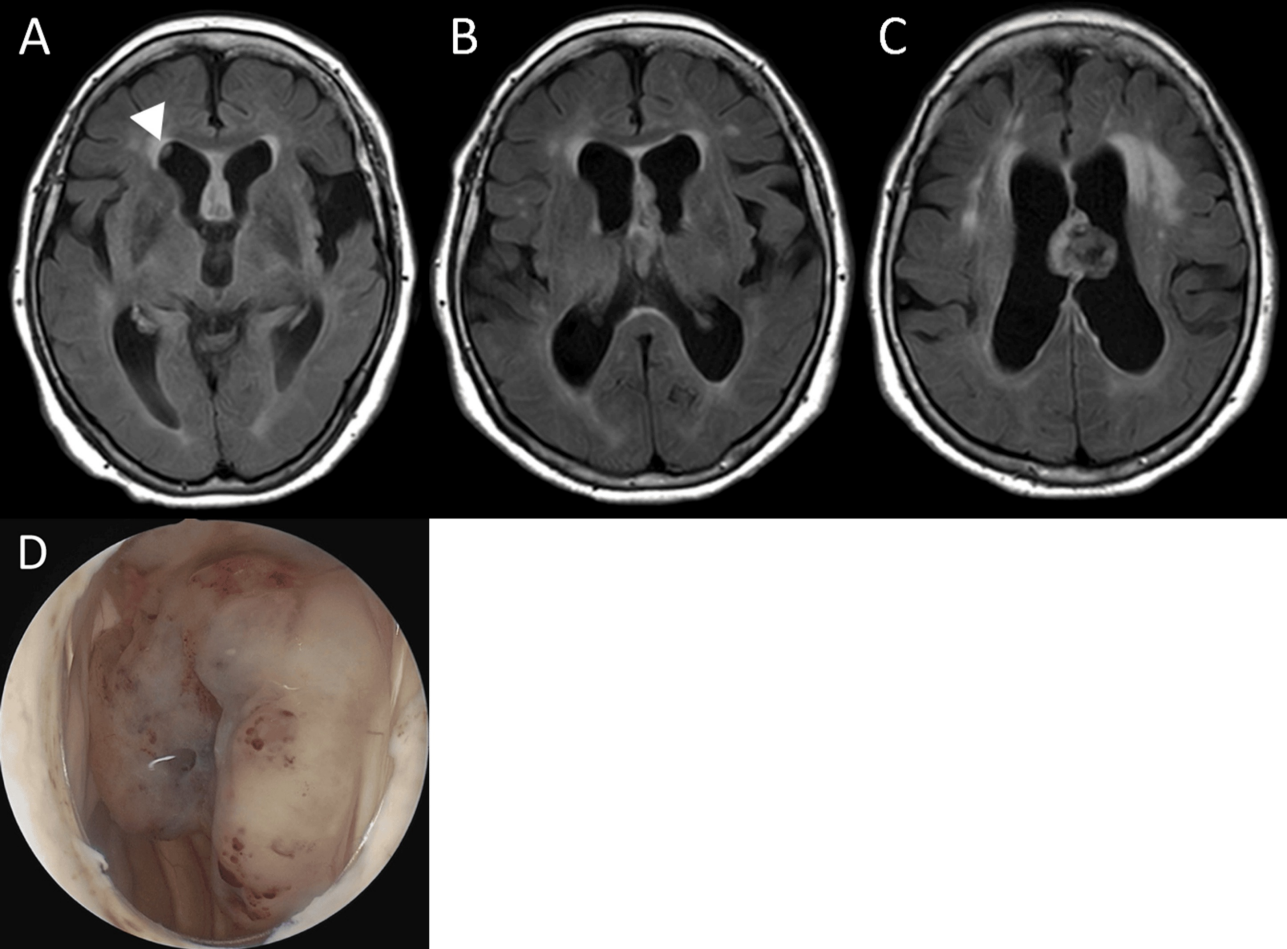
Preoperative MRI and intraoperative endoscopic view. Preoperative MRI (A–C) showing an intraventricular tumor adjacent to the septum pellucidum and extending forward along the median side of the walls of the lateral ventricles, with an isolated small lesion (arrowhead in panel A) inside the right frontal horn. Intraoperative endoscopic view (D) demonstrating a soft pink-tan-colored tumor covered by a fibrous capsule. MRI: magnetic resonance imaging.
